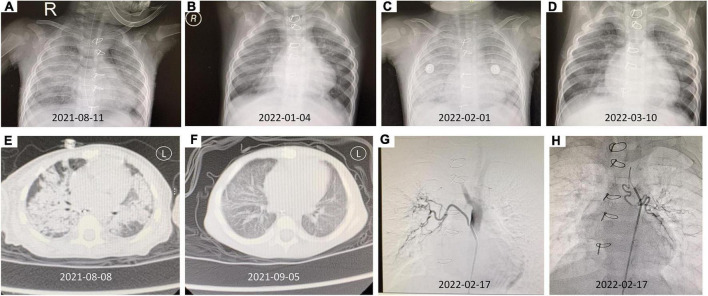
The imaging findings of this patient. (A) Chest X-ray (2021-08-11) showed a massive, diffuse bilateral large pulmonary consolidation. (B) The chest X-ray after therapy (2022-01-04). (C) The chest X-ray (2022-02-01) suggested extensive exudate. (D) After abnormal bronchial artery was embolized, the follow-up chest X-ray (2022-03-10) was significantly better than before. (E) Chest CT (2021-08-08) revealed increased patchy and flaky shadows were evident in both lungs, and a local thickening of bilateral pleura was seen. (F) The chest CT after therapy showed better than Figure 3E (2021-09-05). (G) The DSA showed right bronchial artery originated from the thoracic aorta. (H) The DSA showed left bronchial artery originating from the aortic arch.
